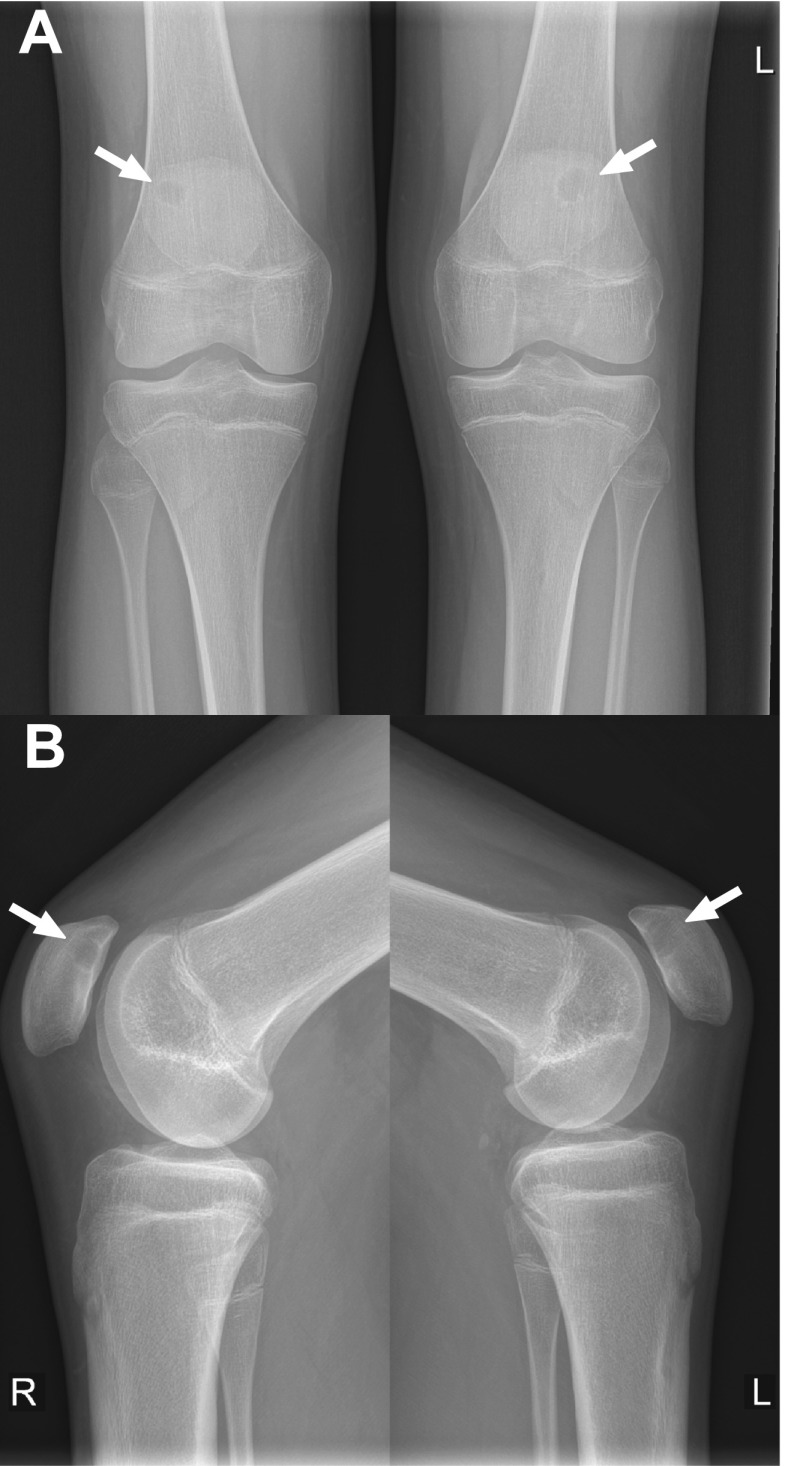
Frontal (a) and lateral (b) radiographs of both knees demonstrate a lytic and round lesion with well-defined margins in the subchondral regions of the superolateral quadrant of both patellae (arrows)
